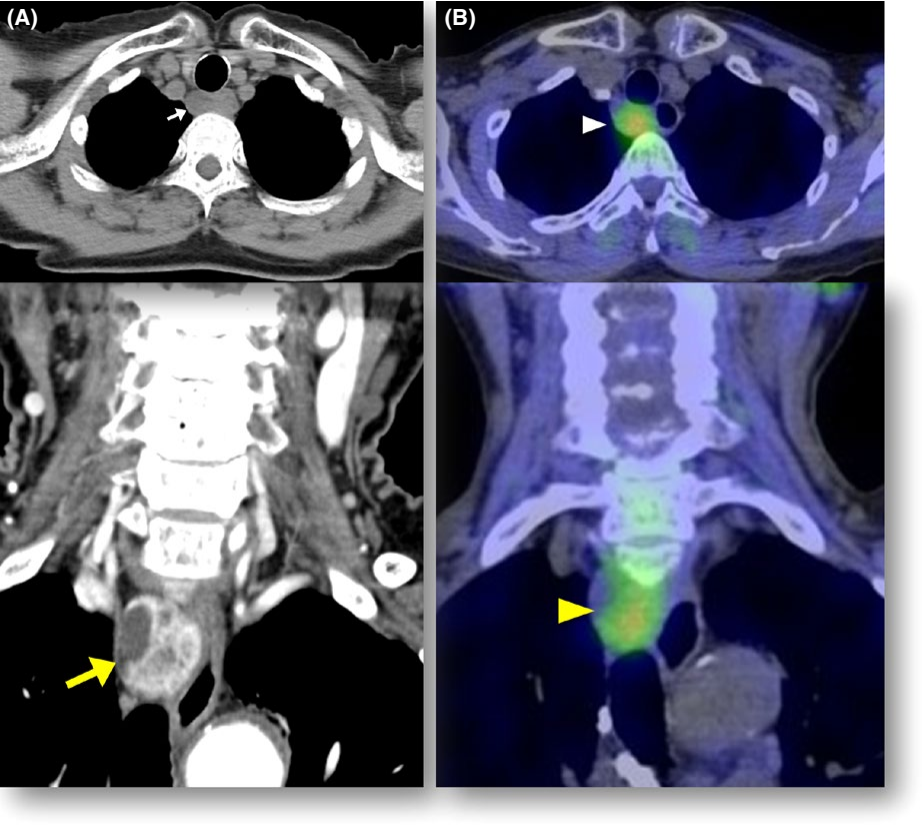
Horizontal view of noncontrast computed tomography (CT) of the mass (A, arrow). Contrast CT demonstrated enhancing mass measuring 2 cm in diameter in the posterior mediastinum (A, yellow arrow). ^131^I‐MIBI SPECT showed uptake in the mass (B, arrowheads)
